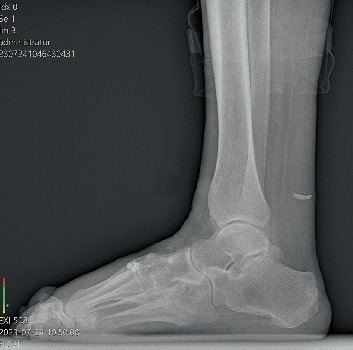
Eight-year postoperative x-ray demonstrating retained anatomic alignment.
